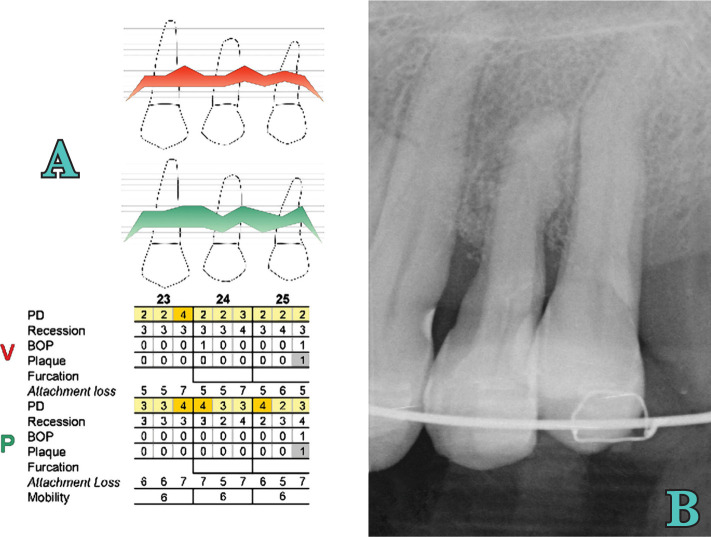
Periodontal (A) and radiographic (B) examination at 4 years.
